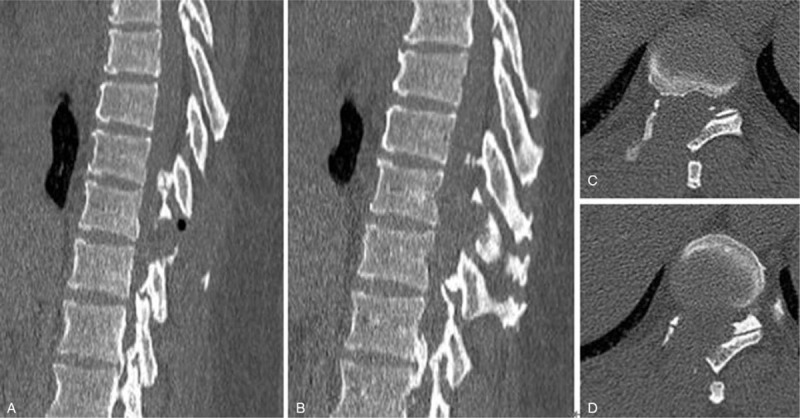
CT on the first day after surgery: Complete resection of ossification of the ligamentum flavum and dural ossification.
